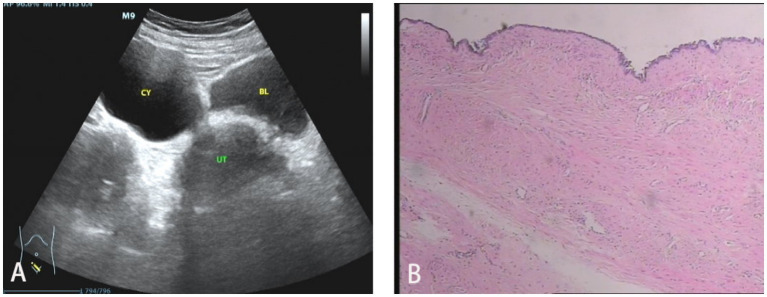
(A) Ultrasonography image (UT: Uterus; BL: Bladder; CY: Cyst) and (B) postoperative pathological result of the first patient.
